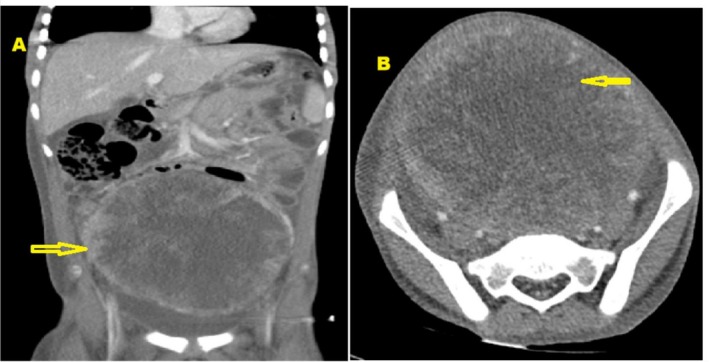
Abdominal CT scan with contrast (A) coronal and (B) axial view revealed a large, heterogeneous, enhancing retroperitoneal mass with central necrosis, measuring 13 × 13 × 10 cm. The mass displaced the intestines superolaterally, compressed the urinary bladder inferiorly, and displaced the ureters laterally, resulting in bilateral hydroureteronephrosis.
